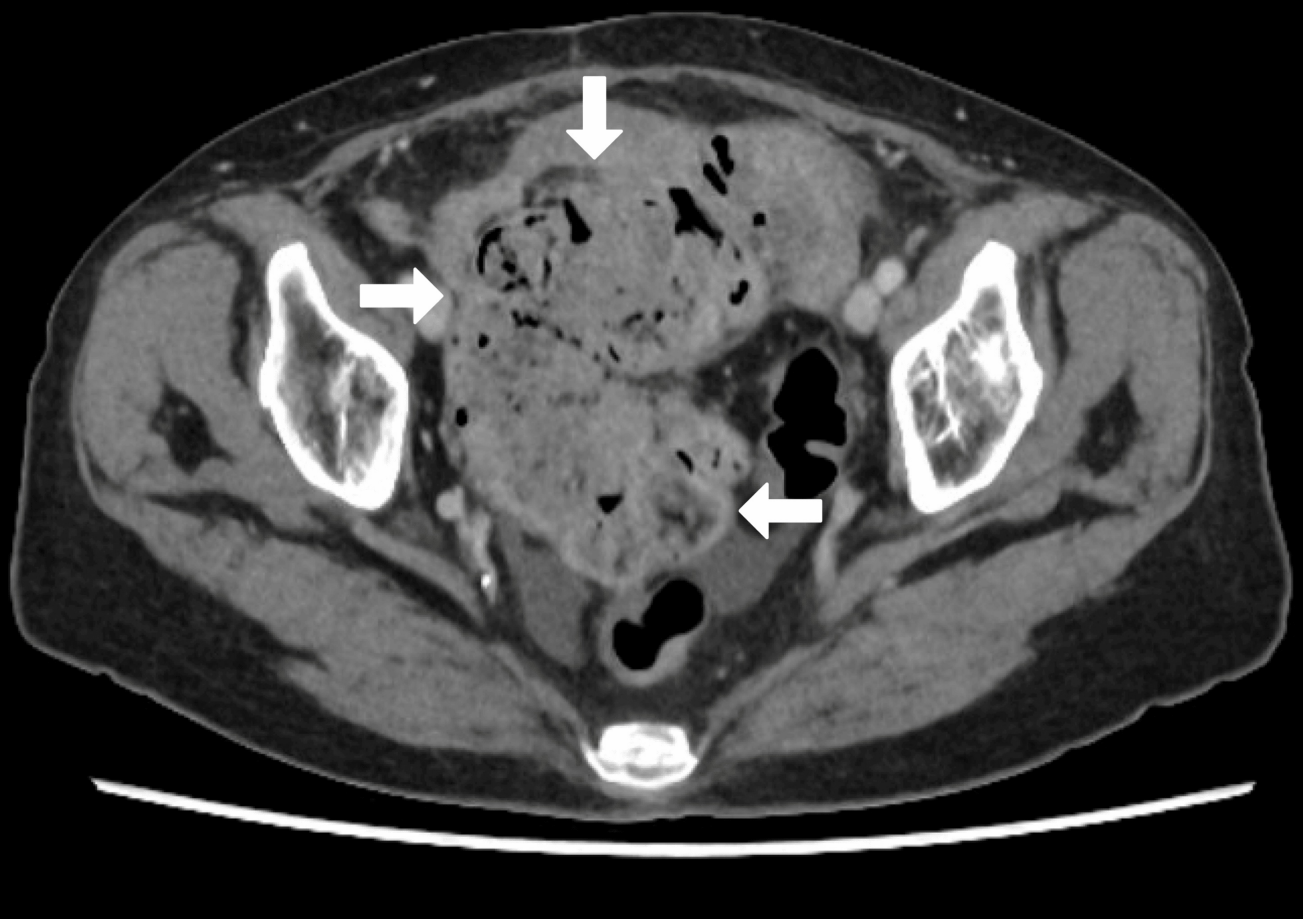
CT Abdomen & Pelvis (Axial View) Perforated sigmoid colon just above the promontory with limited contamination in pelvis, the sigmoid colon severely inflamed and full of hard stool with a 2 cm necrotic patch at the mid sigmoid, site of perforation.
